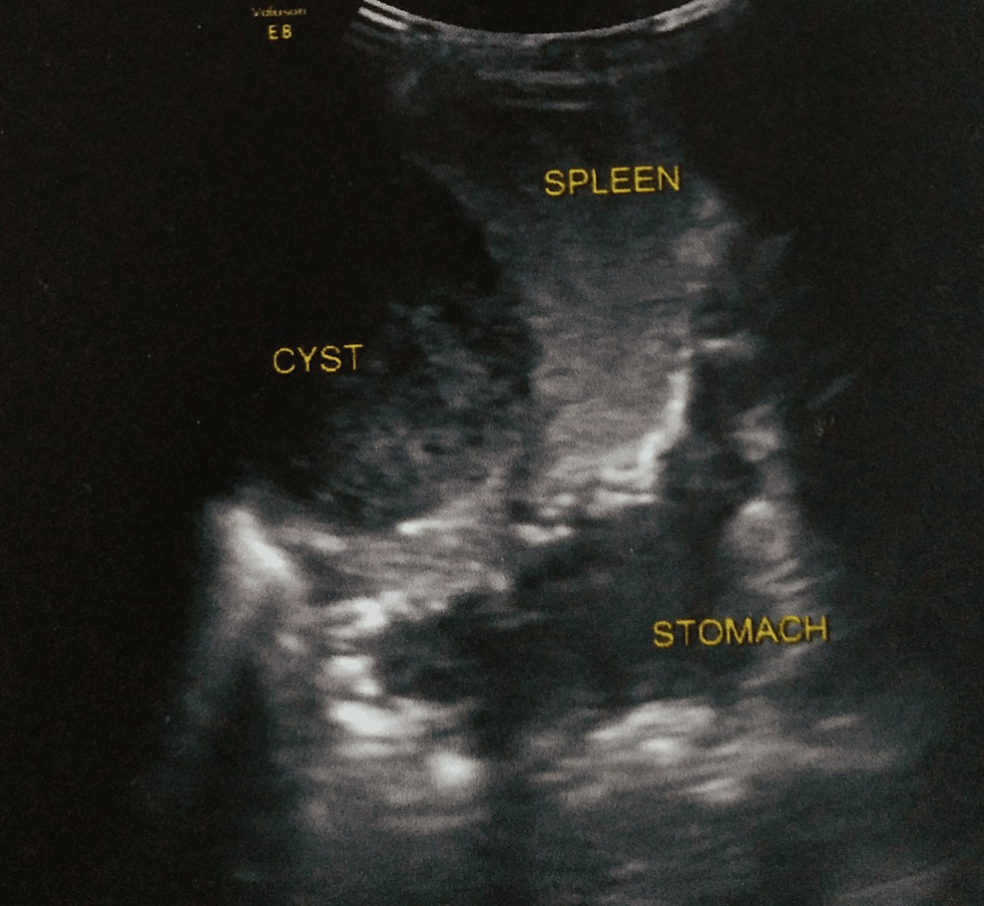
USG of the abdomen showing a well-defined cystic lesion USG: ultrasonography
